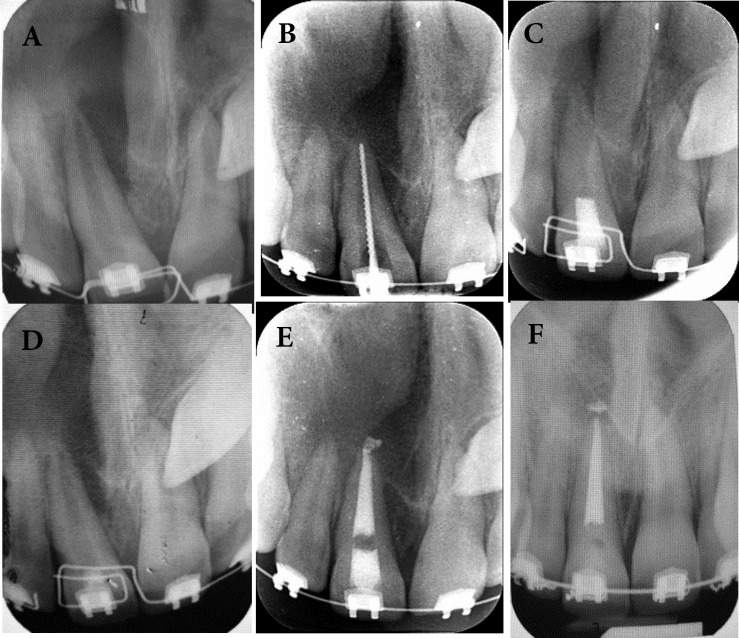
A)* Pretreatment periapical view; *B)* Working radiography; *C)* Application of calcium hydroxide; *D)* After 6 months of calcium hydroxide therapy; *E)* Post treatment radiography; *F)* Follow-up radiography after 20 months
